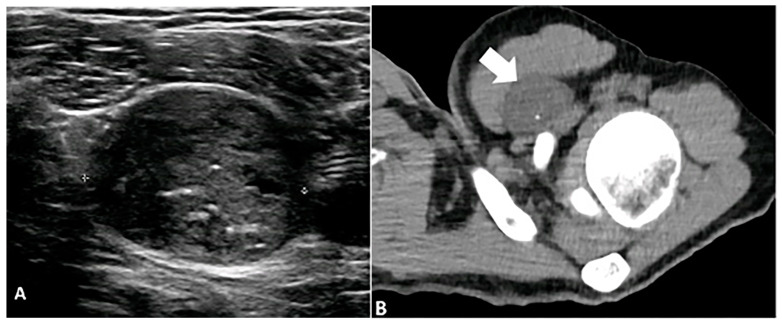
A 23-year-old male with a left subpectoral mass. (A) Ultrasound shows a well-circumscribed, hypoechoic soft tissue mass with internal echogenic foci, representative of punctate calcifications. The mass demonstrates water density (arrow) on CT (B) with punctate calcifications. Subsequent biopsy revealed a benign nerve sheath tumor, schwannoma. The image was obtained from the University of Washington Medical Imaging database with the required permissions.
